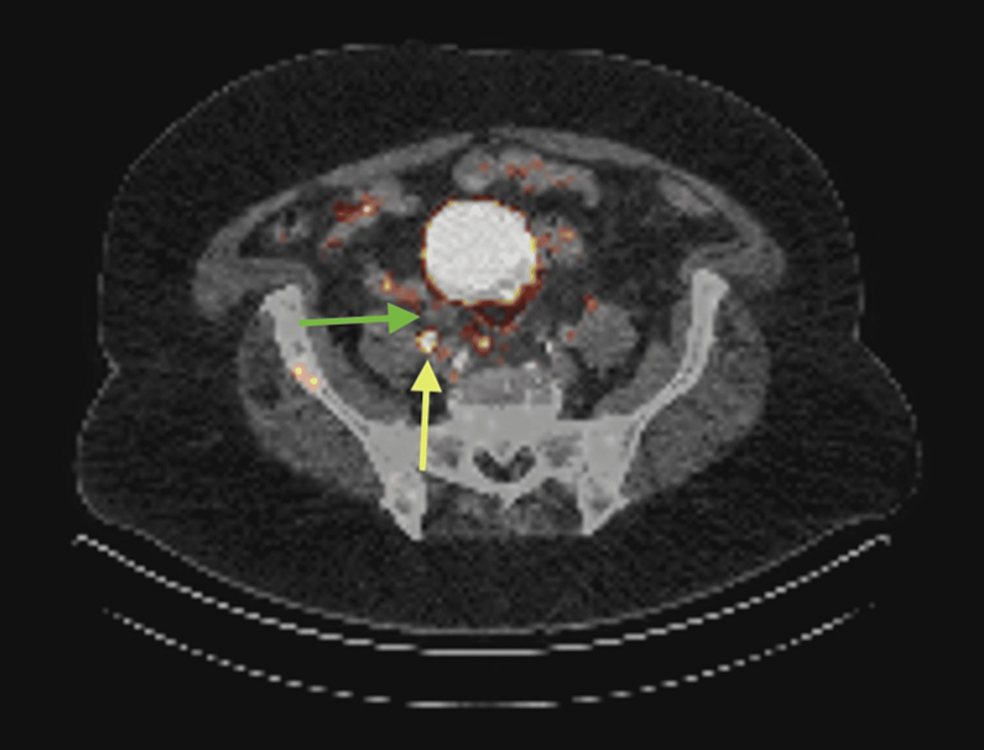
Case II prostate-specific membrane antigen (PSMA) positron emission tomography (PET) scan The yellow arrow here shows metastatic adenopathy in the retroperitoneum and pelvis. The green arrow highlights right hydroureteronephrosis to the distal ureter
